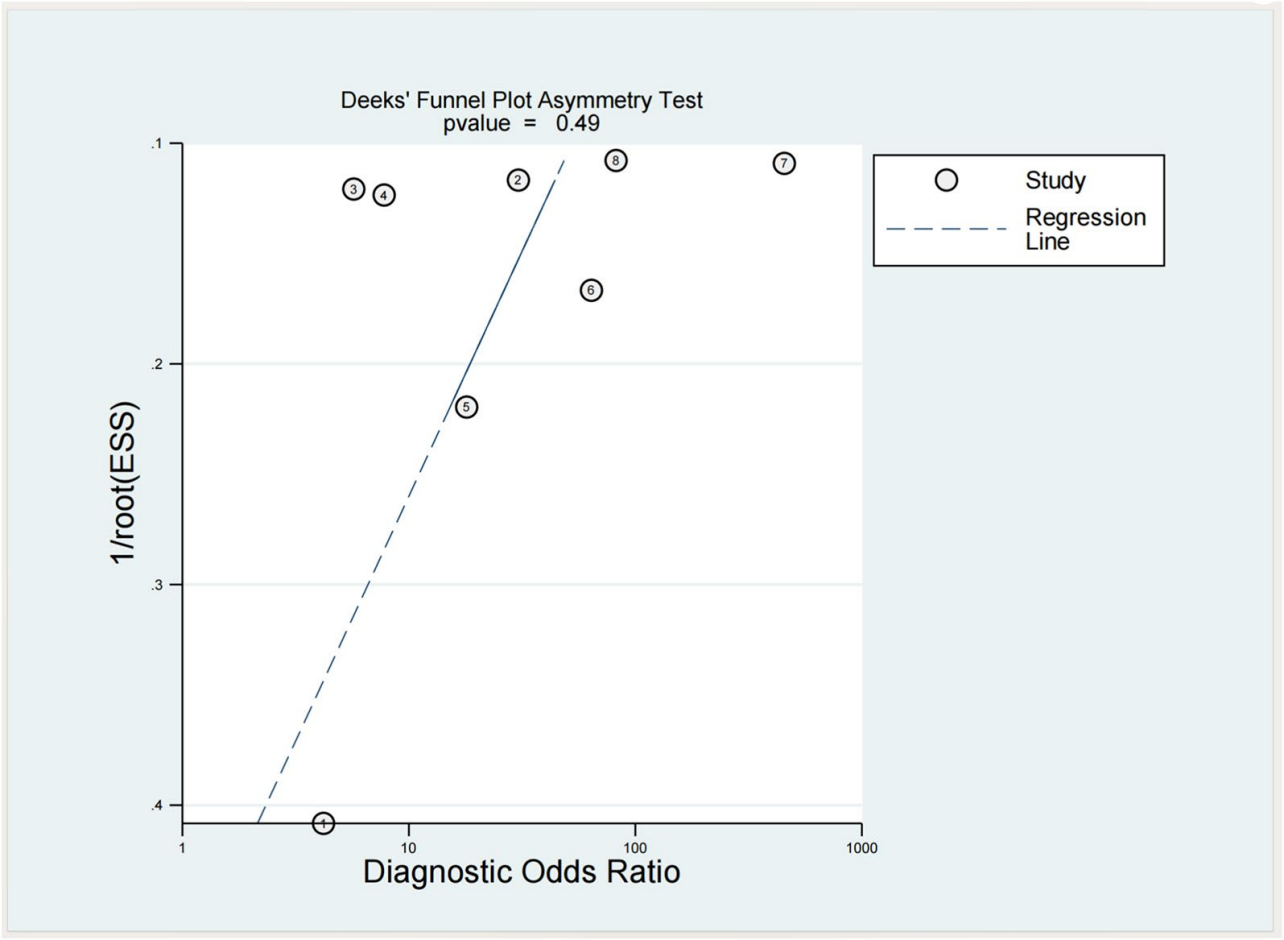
Deeks’ funnel plot asymmetry test for assessing publication bias in ultrasound-related dysphagia studies.
